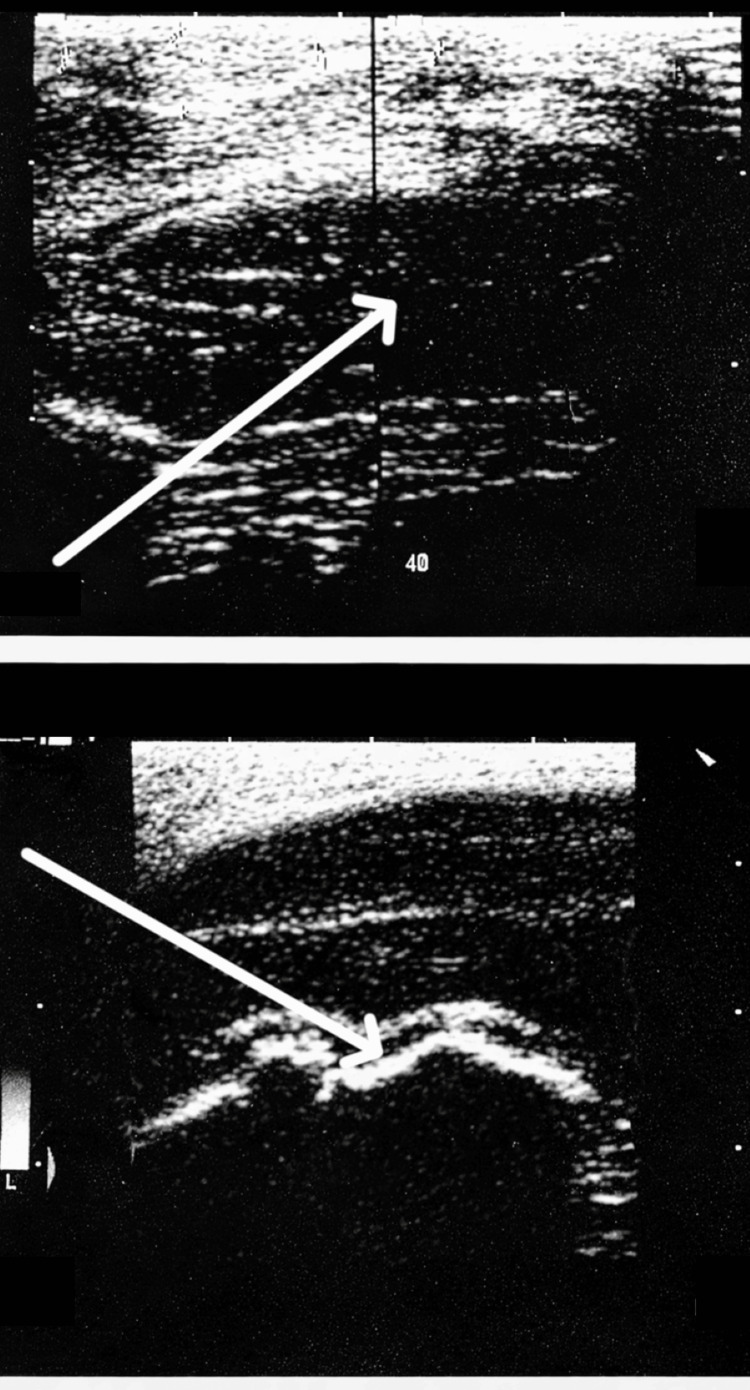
Ultrasound image with arrow showing the lesion
